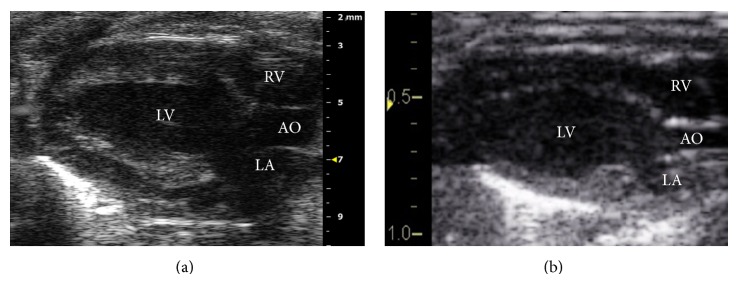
2D greyscale of parasternal long axis acquired by (a) VSI Vevo 2100 and (b) GE Vivid 7. LV: left ventricle; RV: right ventricle; LA: left atrium; AO: aorta.
